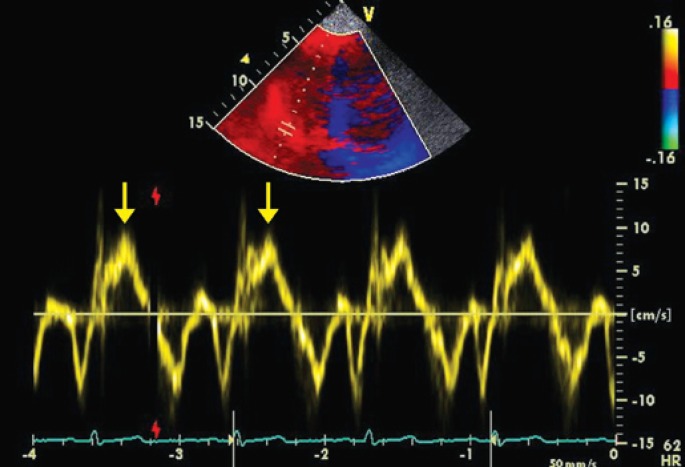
Tissue Doppler of the tricuspid annulus showing decreased (7cm/s) systolic velocity (s') in a patient with pulmonary thromboembolism and moderate right ventricular dysfunction (yellow arrows)
